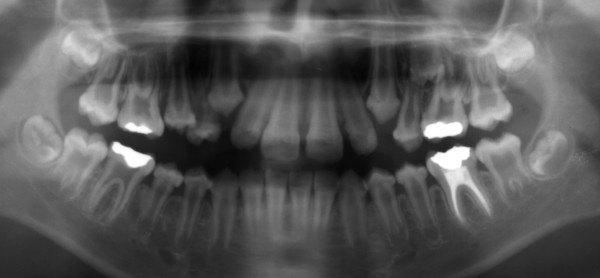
Patient 1: Panoramic radiograph at 12 years of age. The radiograph shows enlarged pulp chambers and abnormality of the shape of crown form. The second molars present more severe taurodontism than the first molars.
